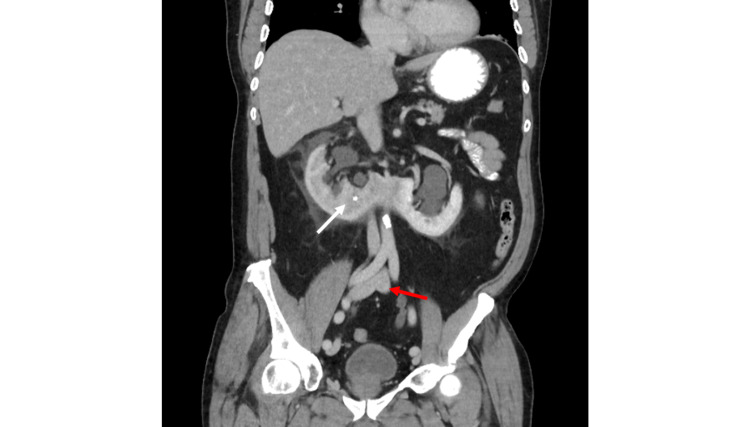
Coronal contrast-enhanced CT with intravenous and oral contrast demonstrates horseshoe kidney with nephrolithiasis bilaterally (white arrow). Of note, the iliac vein confluence is at the pelvic inlet, which is at the level of the S1 vertebral body (red arrow).
